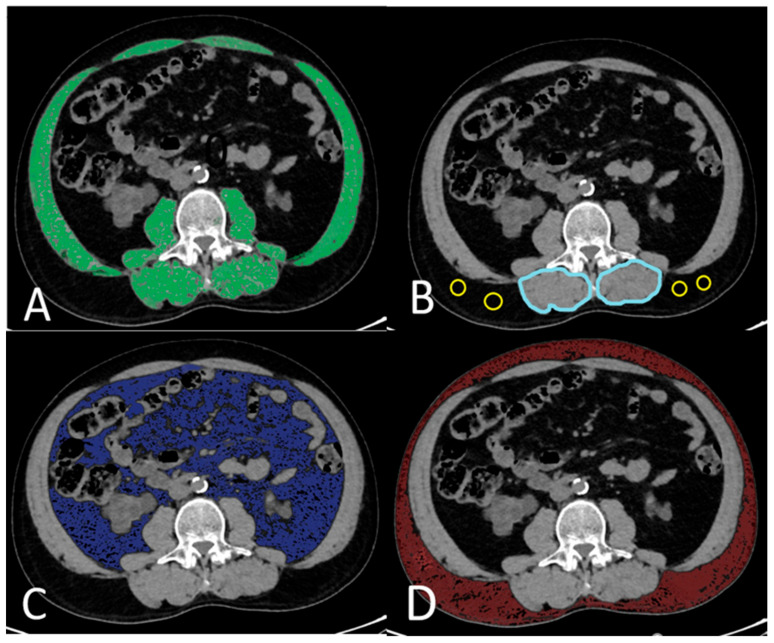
Cross-sectional computed tomographic images obtained at the L3 vertebral level. (A), SMA (green-painted area), including psoas, erector spinae, quadratus lumborum, transversus abdominis, external and internal obliques, and rectus abdominis, were identified and measured using −29 to 150 HU. (B), CT values of subfascial muscle tissue (2 large blue circles) and subcutaneous fat (4 small circles) in the multifidus muscle are examined to calculate IMAC. (C), VAT areas (blue-painted area) measured using −150 to −50 HU. (D), Subcutaneous adipose tissue areas (red-painted area) were measured using −190 to −30 HU. HU, Hounsfield units, SMA: Skeletal muscle area, VAT: Visceral adipose tissue.
